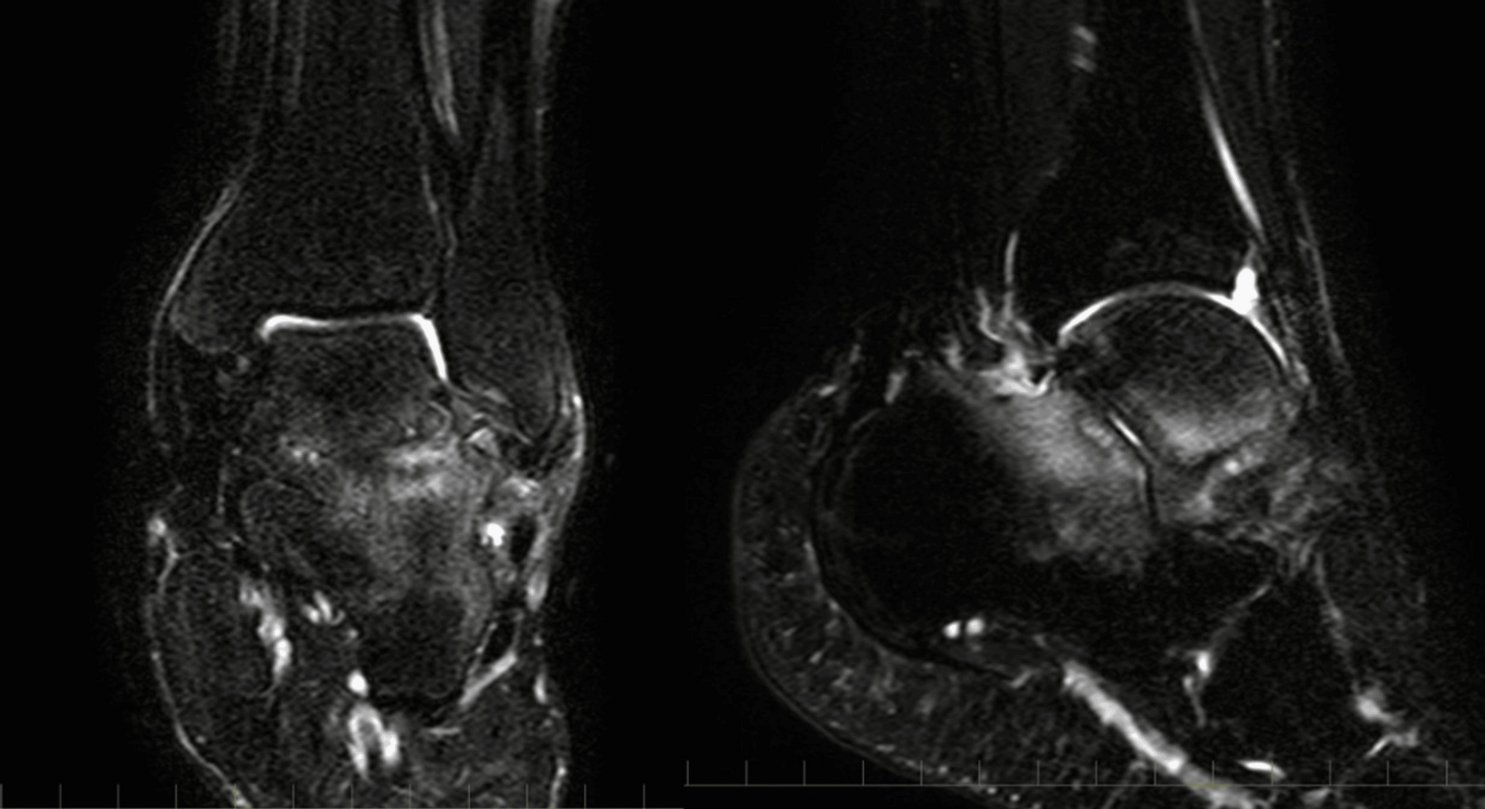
MRI finding. MRI shows a bone marrow lesion area at the subtalar joint.
